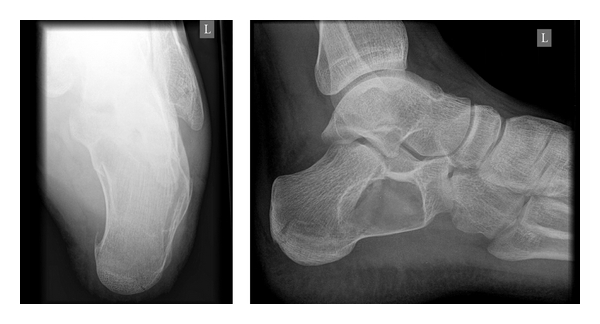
Axial and lateral plain X-rays showing a large radiolucent area in the anterior half of the calcaneal body resembling to a cyst. The lines of the pathologic fracture can also be observed.
